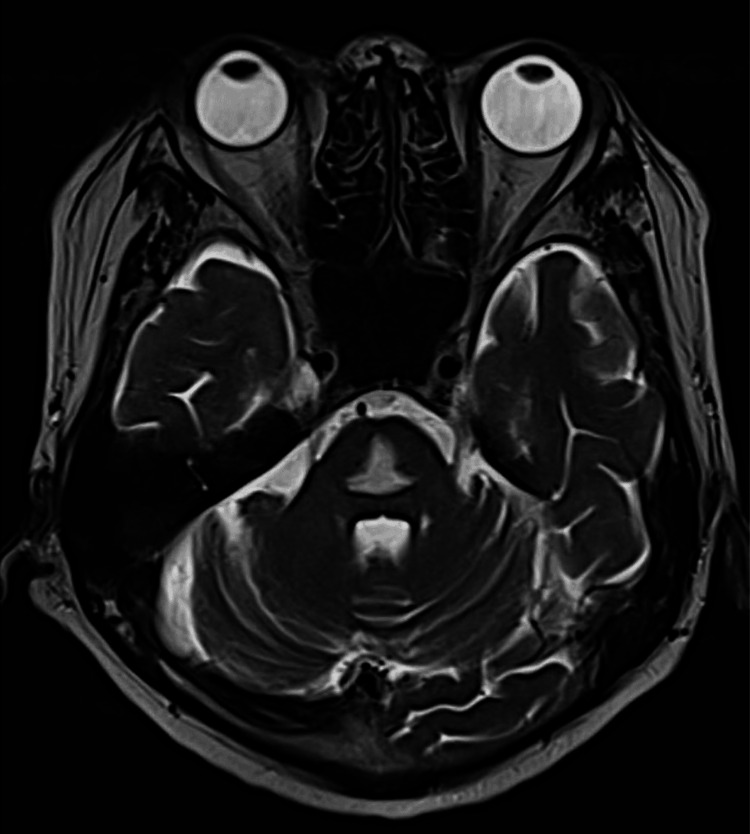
Axial T2-weighted MRI of the brain showing a symmetric hyperintense lesion in the central pons with a characteristic trident-shaped appearance, consistent with ODS ODS, osmotic demyelination syndrome
